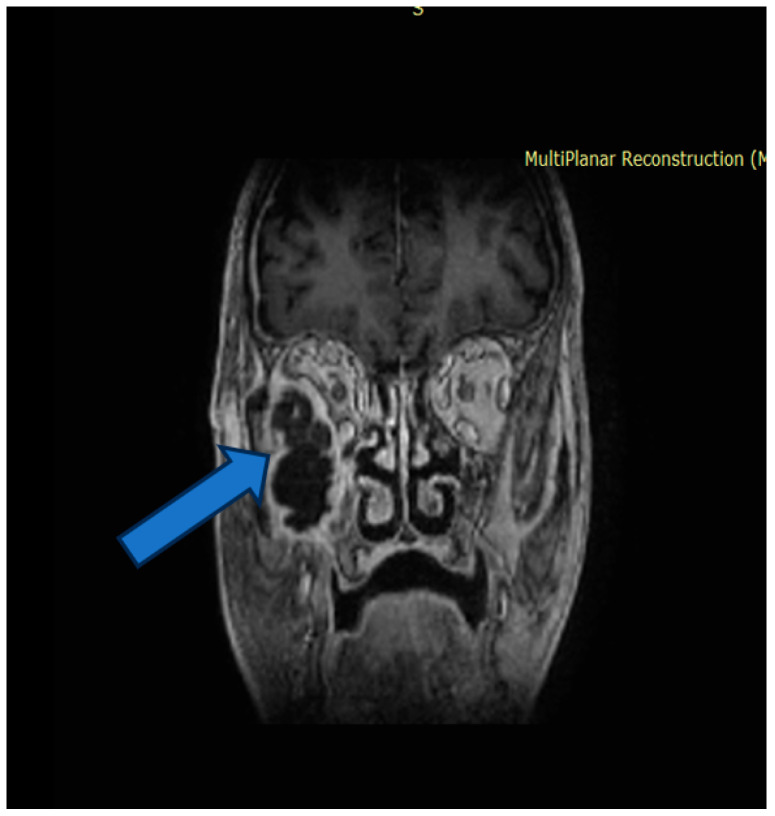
Magnetic resonance imaging before surgery. A mass-like structure with cystic and parenchymatous components. Invasion of the tumor in the right orbit (marked with arrow).
